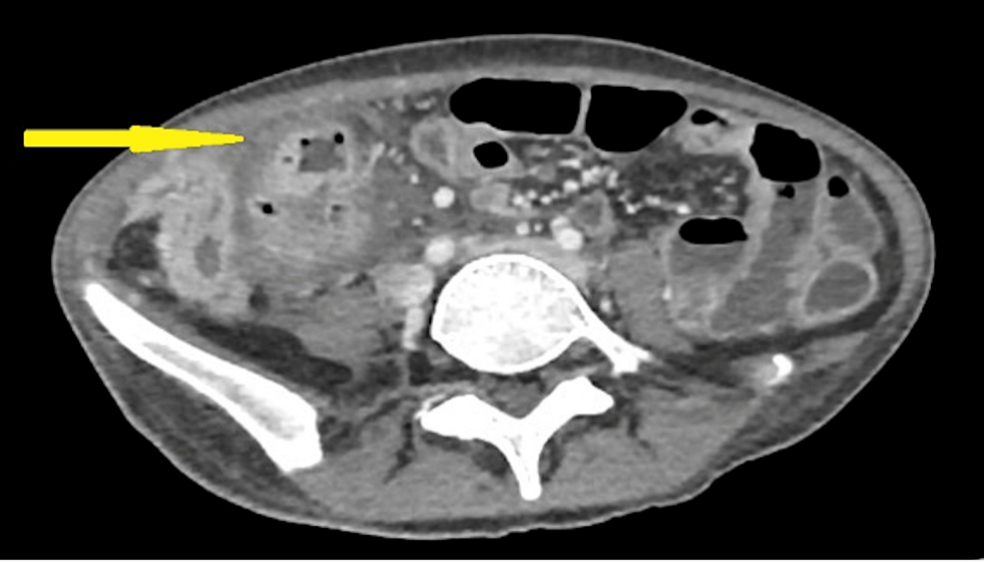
CT scan (Axial cut) showing a heterogenous enhancing collection (yellow arrow) at the right paracolic gutter
